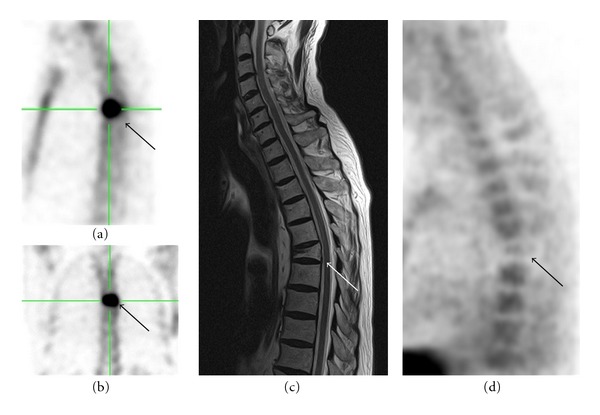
((a), (b)) Bone scan, sagittal and coronal planes, respectively, show an intensive uptake in middle thoracic spine. (c) MRI-T1 weighted shows a compression fracture at the same location. (d) ^18^F-FECH-PET shows relatively an uptake reduction.
